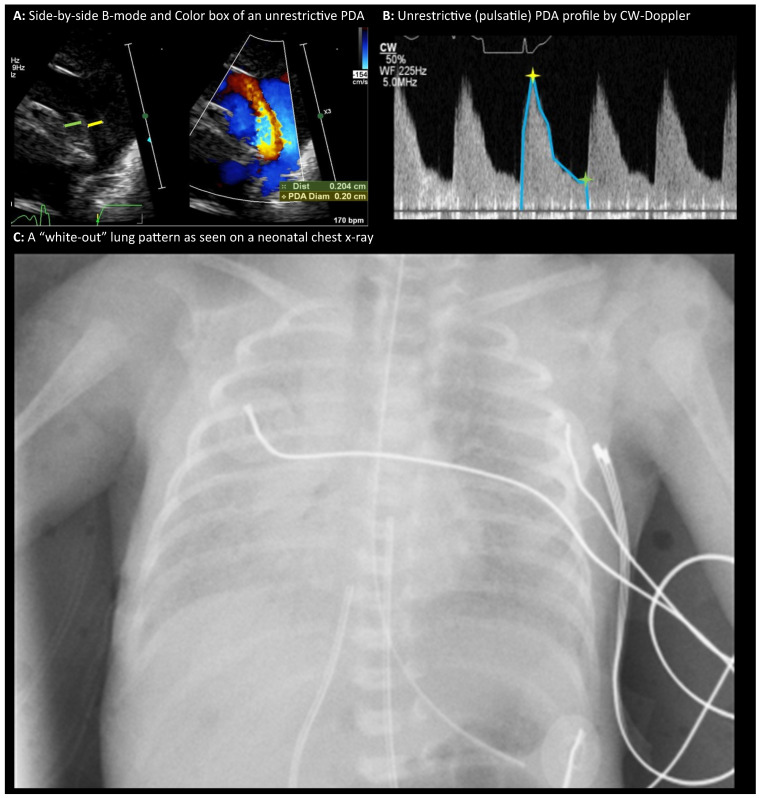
Diagnostic Imaging Profile of a Neonate With a 0.20 cm Unrestrictive PDA and “White-Out” Lung Pattern (A) Side-by-side B-mode and color box of an unrestrictive PDA with a 0.20 cm ductal diameter (in yellow) and left-to-right shunt (the left pulmonary artery is also measured alongside in green (0.204 cm) for comparison). (B) CW-Doppler profile of an unrestrictive PDA showing a pulsatile waveform with a sharp systolic peak (yellow star) and a diastolic decline (green star). (C) A “white-out” lung pattern as seen on a neonatal chest x-ray. Abbreviations: PDA (patent ductus arteriosus); CW (continuous wave); B-mode (brightness mode); cm (centimeters); bpm (beats per minute); MHz (megahertz); Hz (hertz).
